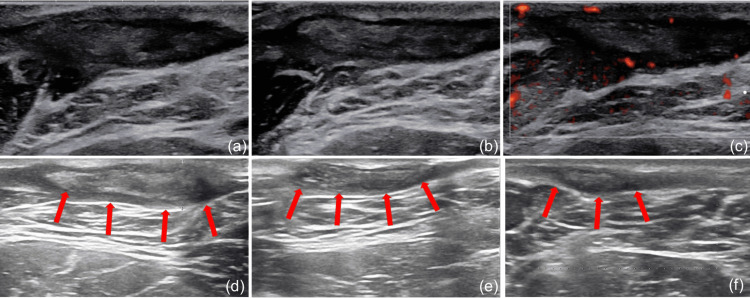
Ultrasonic follow-up after anti-TB treatment August 17, 2023 (a-c): The subcutaneous soft tissue of the left neck presents a patchy inhomogeneous hypoechoic area with a range of approximately 3.6 × 0.9 cm and increased blood flow signals. November 23, 2023 (d): An irregular strip-shaped hypoechoic area is visible under the skin of the left neck, with a thickness of approximately 0.7 cm, and no obvious blood flow signals are observed within it. March 28, 2024 (e, f): An irregular strip-shaped hypoechoic area is visible under the skin of the left neck, with a thickness of approximately 0.45 cm, and no obvious blood flow signals are observed within it. TB: tuberculosis
